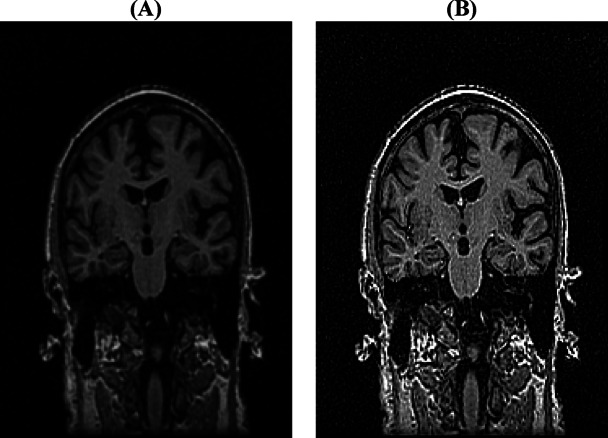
The preprocessed image dataset from the sample MRI images sourced from the Alzheimer’s disease neuroimaging initiative (ADNI) dataset: (a) Input image; (b) Filtered image.
